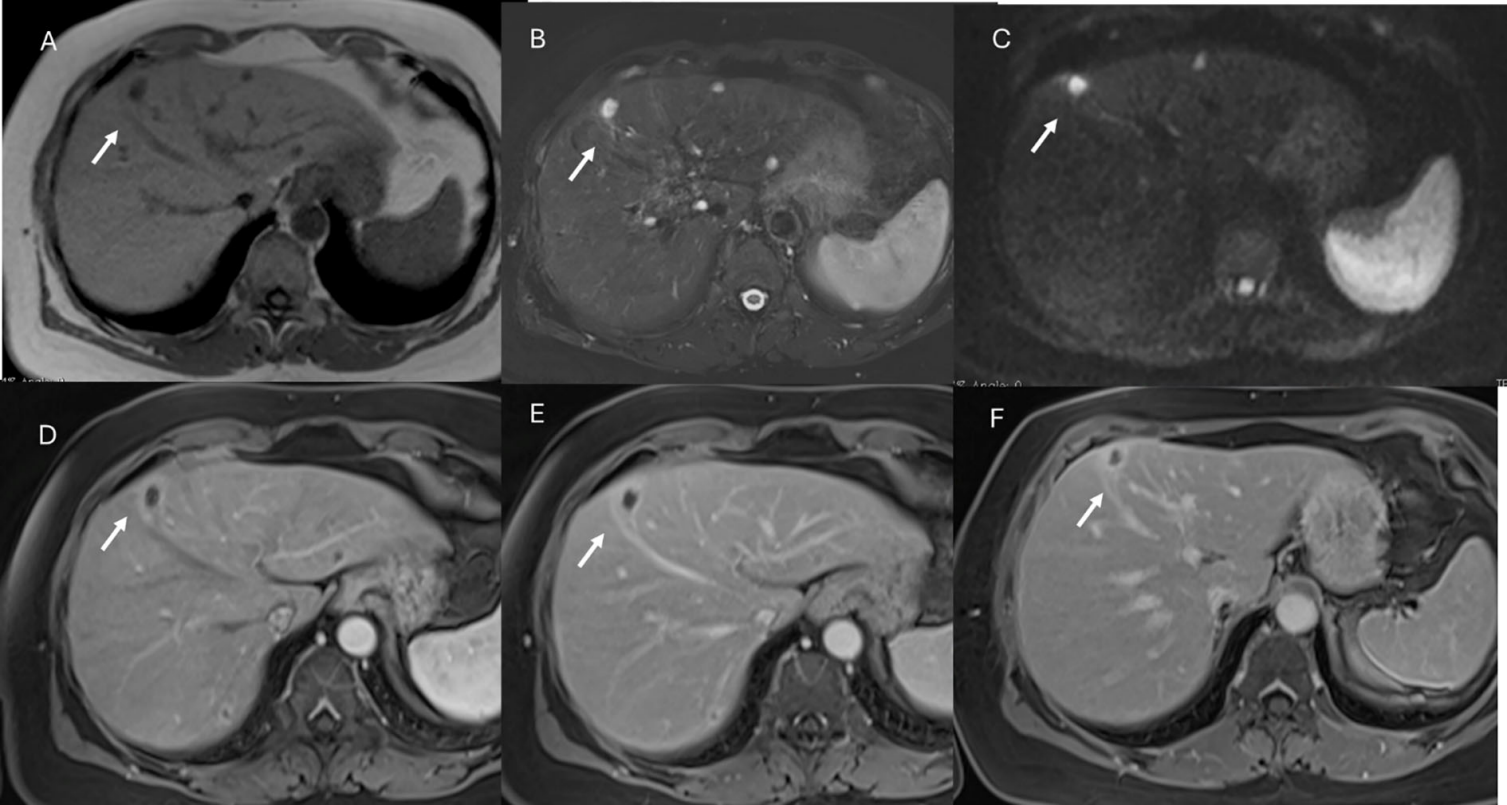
MRI assessment of mucinous colorectal liver metastases (arrows). In (A) (T1-W sequence, axial plane), the lesion (arrow) shows hypointense signal, with very high signal on T2-W FS sequence (B) and restricted diffusion on b = 880 s/mm² (C). After non-specific contrast agent, rim enhancement is seen in the arterial phase (D, T1-W, axial), with progressive enhancement in the portal (E) and late phases (F).
